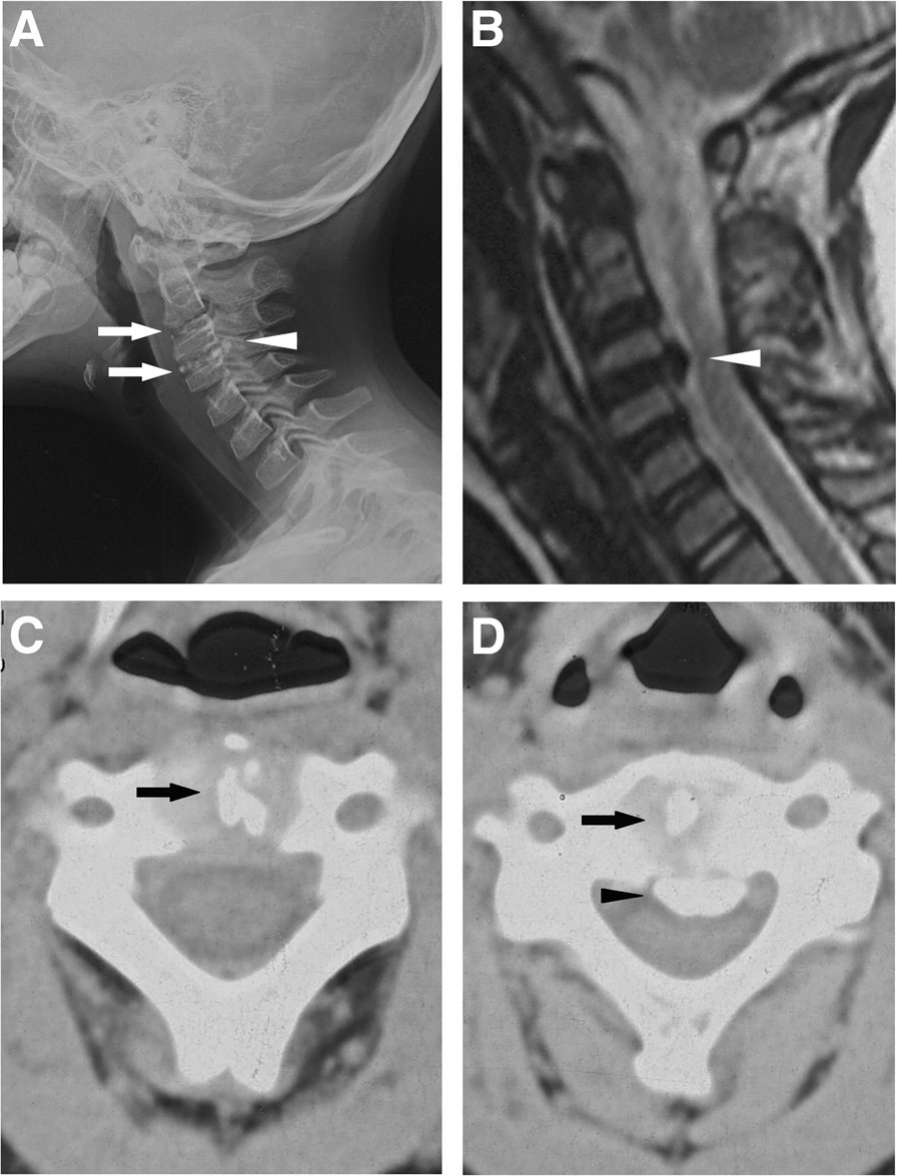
Radiological imaging at admission. a Lateral cervical spine radiograph showed C2/3and C3/4 IDC (thin arrows) and OPLL at C3 and C4 (thick arrow). b Magnetic resonance imaging showed that spinal cord was compressed anteriorly at the C3/4 level (arrow). c Axial computed tomography through C2/3 revealed IDC (thick arrows) at C2/3. d Axial computed tomography through C3/4 revealed IDC (thick arrows) at C3/4 and OPLL at C3/4 level (thin arrow). (Adopted and reedited from Du et al. [3] with permissions of all authors)
